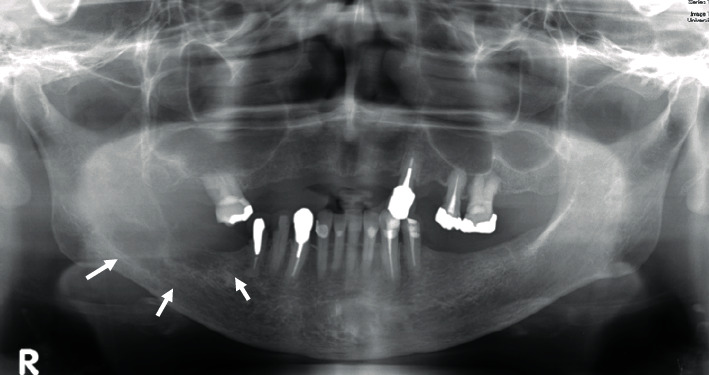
Panoramic radiograph. The panoramic radiograph revealed irregular radiolucent region on the right body to ramus infiltrating to the mandibular canal.
